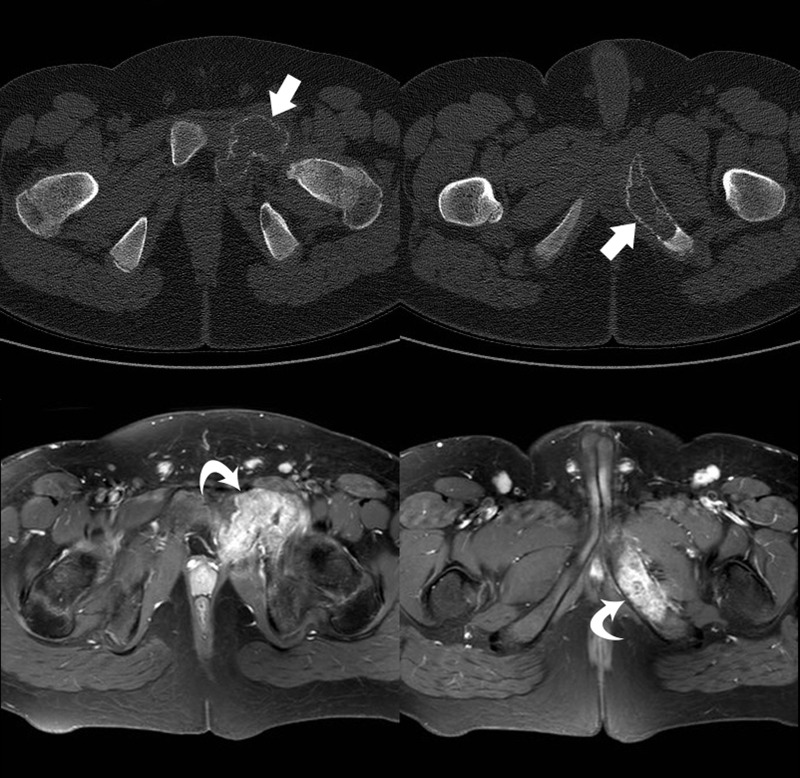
Cross-Sectional Imaging CT axial images (bone window), top row. Gadolinium-enhanced T1-weighted fat-saturated MRI axial images, bottom row. The pelvic lesion demonstrated bony expansion without significant cortical disruption (top row, straight white arrows). The MRI demonstrated heterogeneous enhancement (bottom row, curved white arrows).
